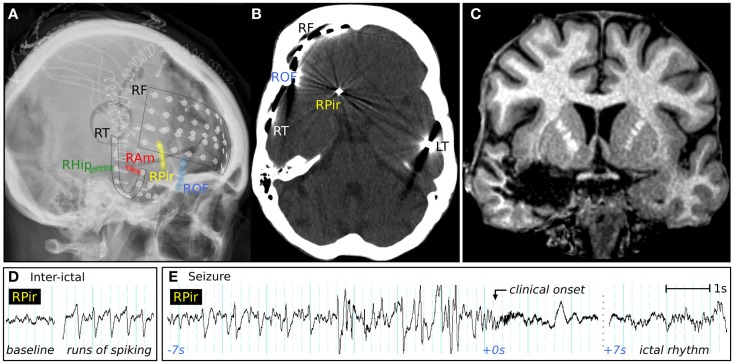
Clinical imaging of a patient with possible piriform epilepsy. (A) Lateral skull X-ray showing positions of intracranial electrodes. RF, right frontal subdural electrodes; RT, right temporal subdural electrode strip; RHip, right hippocampal depth electrode; RAm, right amygdala depth electrodes; RPir, right piriform electrodes; ROF, right orbitofrontal subdural electrodes; LT, left temporal subdural electrode strip. (B) CT performed in the piriform axis showing the position of the most inferomesial RPir electrode contact, in orbitofrontal cortex adjacent to frontal piriform cortex. (C) Coronal T1-weighted MRI, showing posterior extent of surgical resection, with removal of right frontal piriform cortex. (D) EEG recorded from most inferomesial RPir electrode, showing trains of inter-ictal spiking, and (E) a seizure from sleep, with progressively building discharges, then gamma activity and attenuation, followed 7 s later by an evolving ictal rhythm. At the “clinical onset,” there was explosive onset of screaming and flailing movements of the limbs.
